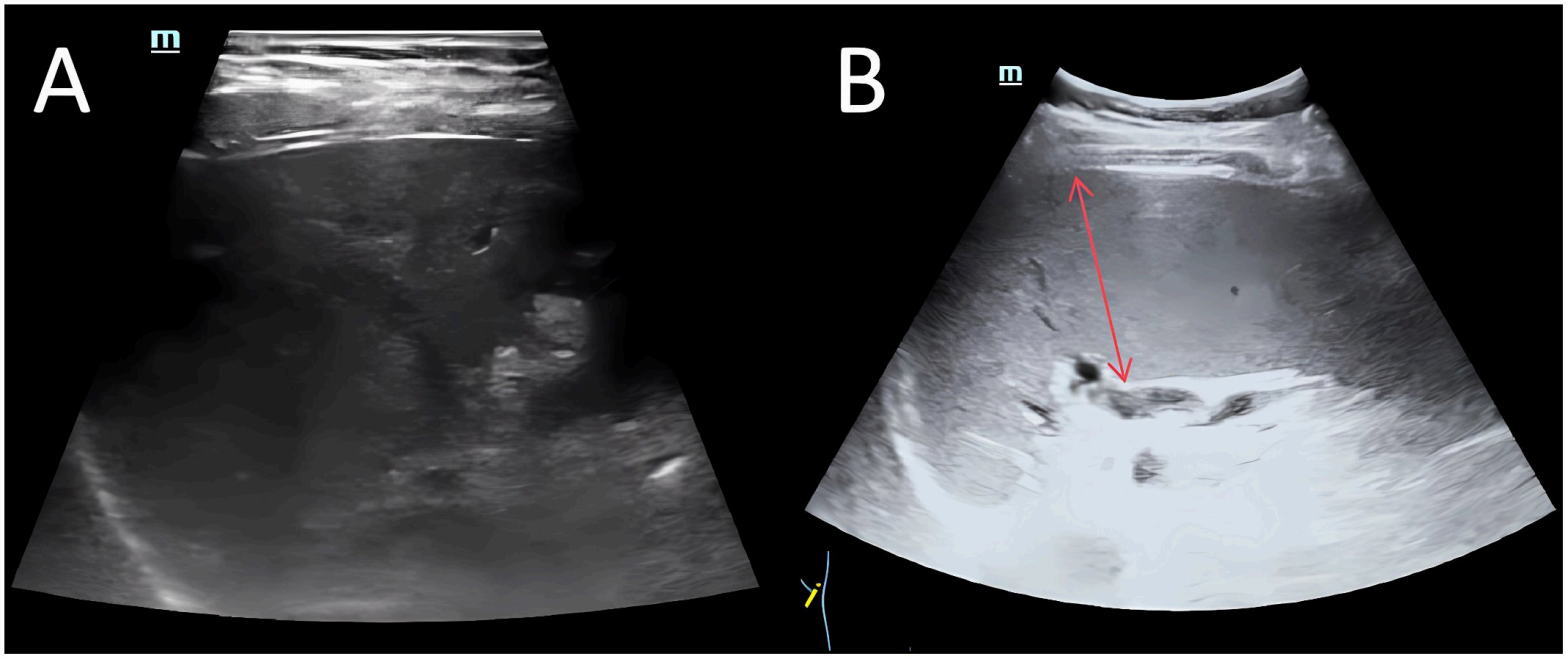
Abdominal ultrasound examination images. (A) Shows coarsened and enhanced hepatic parenchymal echogenicity with uneven distribution, suggesting hepatic parenchymal injury. (B) Demonstrates a splenic intercostal thickness greater than 4 cm, indicating splenomegaly.
